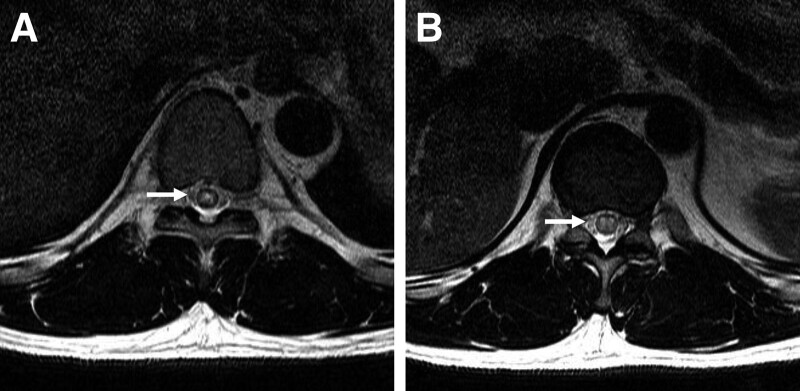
Axial magnetic resonance images at T9 (A) and T11 (B) levels. T2-weighted sequences show the classic “owl’s eye” pattern within the gray matter.
